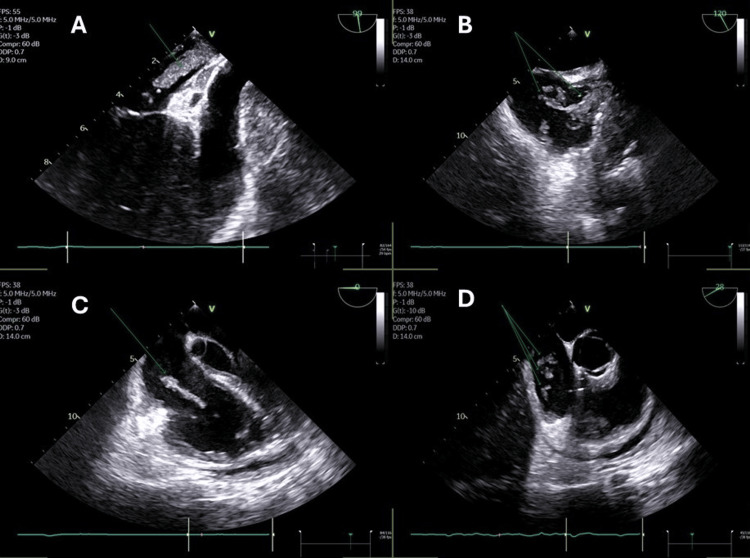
Transesophageal echocardiography (TEE) showing mass origins and mobility A: Mid-esophageal 90-degree view (pulmonary vein adjusted). Single green arrow identifies the left atrial mass protruding from the left superior pulmonary vein. B: Mid-esophageal bicaval 120-degree view (superior vena cava adjusted). Double green arrows show the right atrial mass emerging from the superior vena cava. C: Mid-esophageal 0-degree view. Single green arrow demonstrates the right atrial mass prolapsing from the right atrium into the right ventricle across the tricuspid valve. D: Mid-esophageal 28-degree view. Triple green arrows indicate the right atrial mass within the right atrial chamber.
